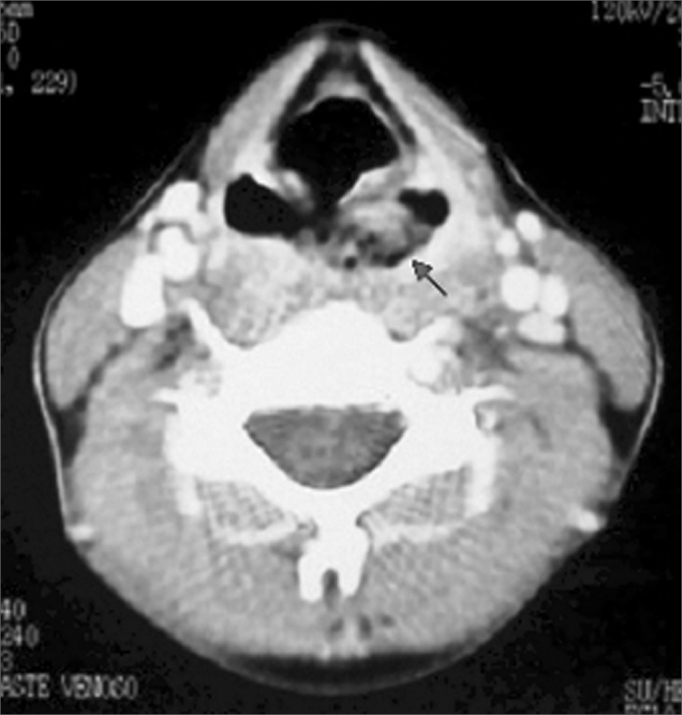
Neck CT scan, axial view: tumor mass in the left piriform cavity.
